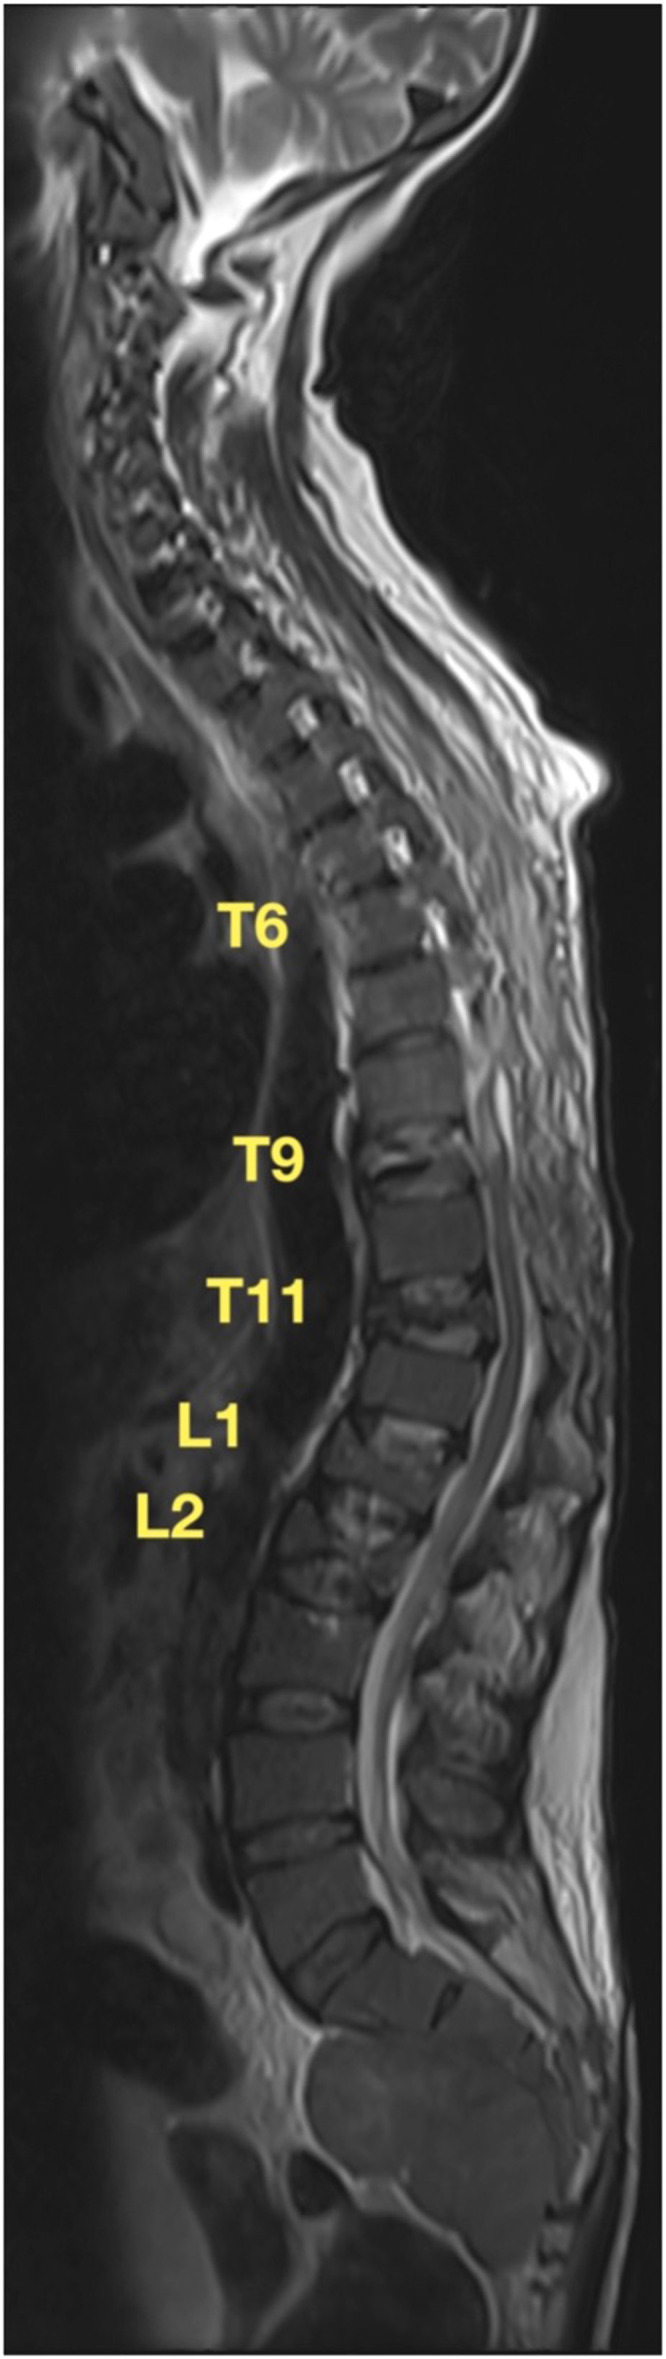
Immediate postoperative MRI demonstrating decompressed thoracic spinal cord with no residual dorsal extradural masses.
